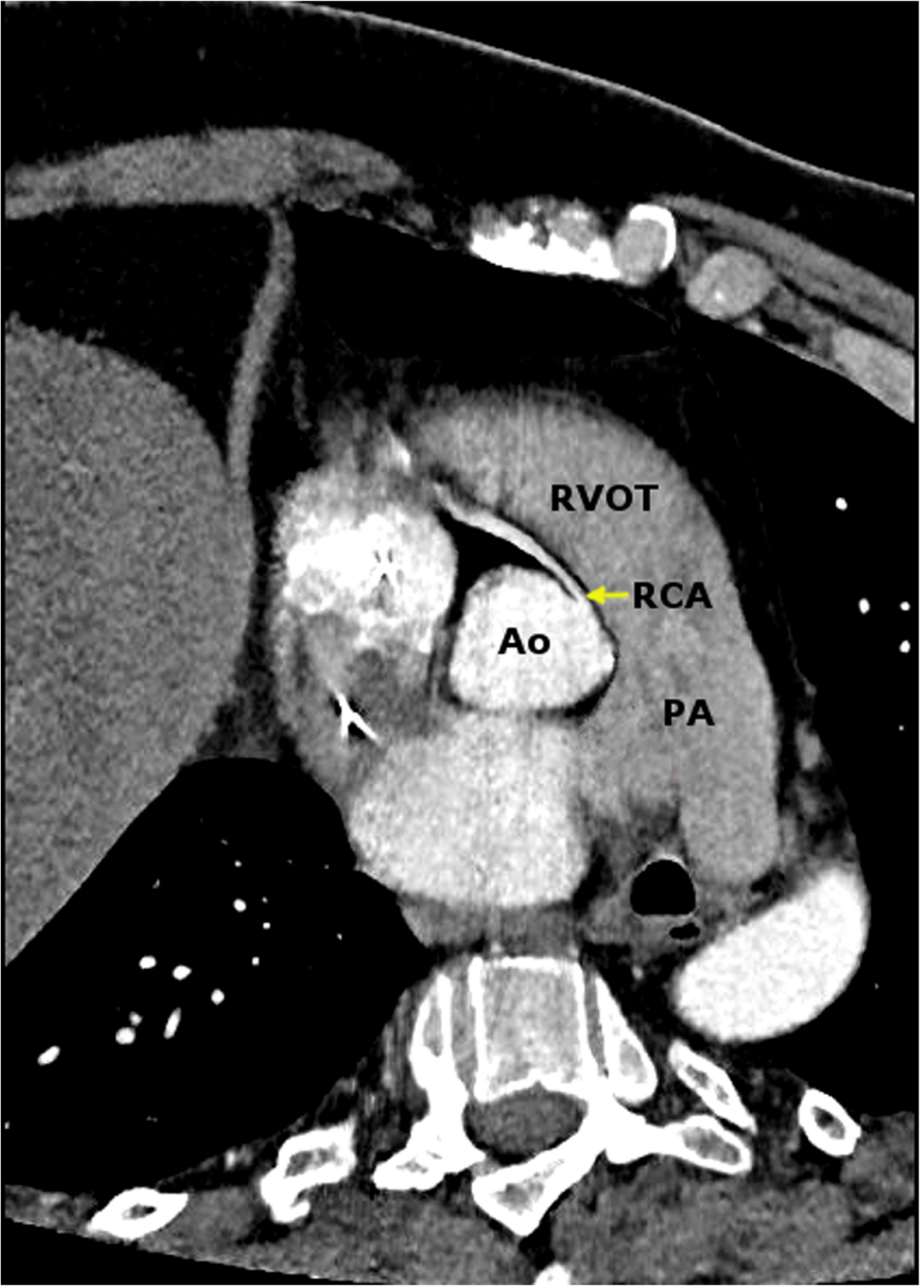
Computed tomographic angiogram indicating compression of RCA between the great vessels at its origin. (PA-Pulmonary artery, RVOT- Right ventricular outflow tract, Ao-Aorta)
